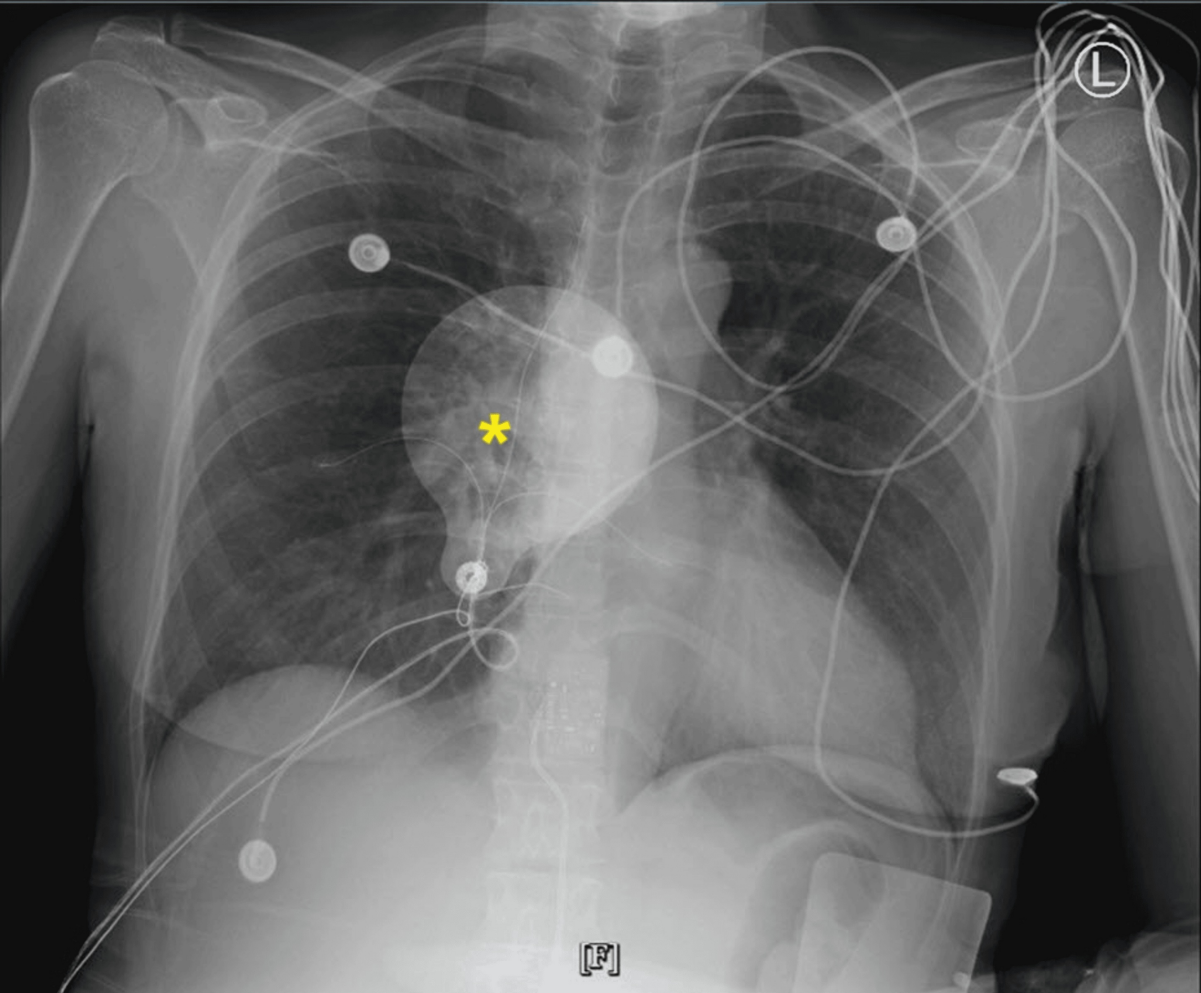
Chest X-ray on admission No focal pulmonary consolidations or significant pleural effusions. The asterisk (*) denotes the external defibrillator pad.
